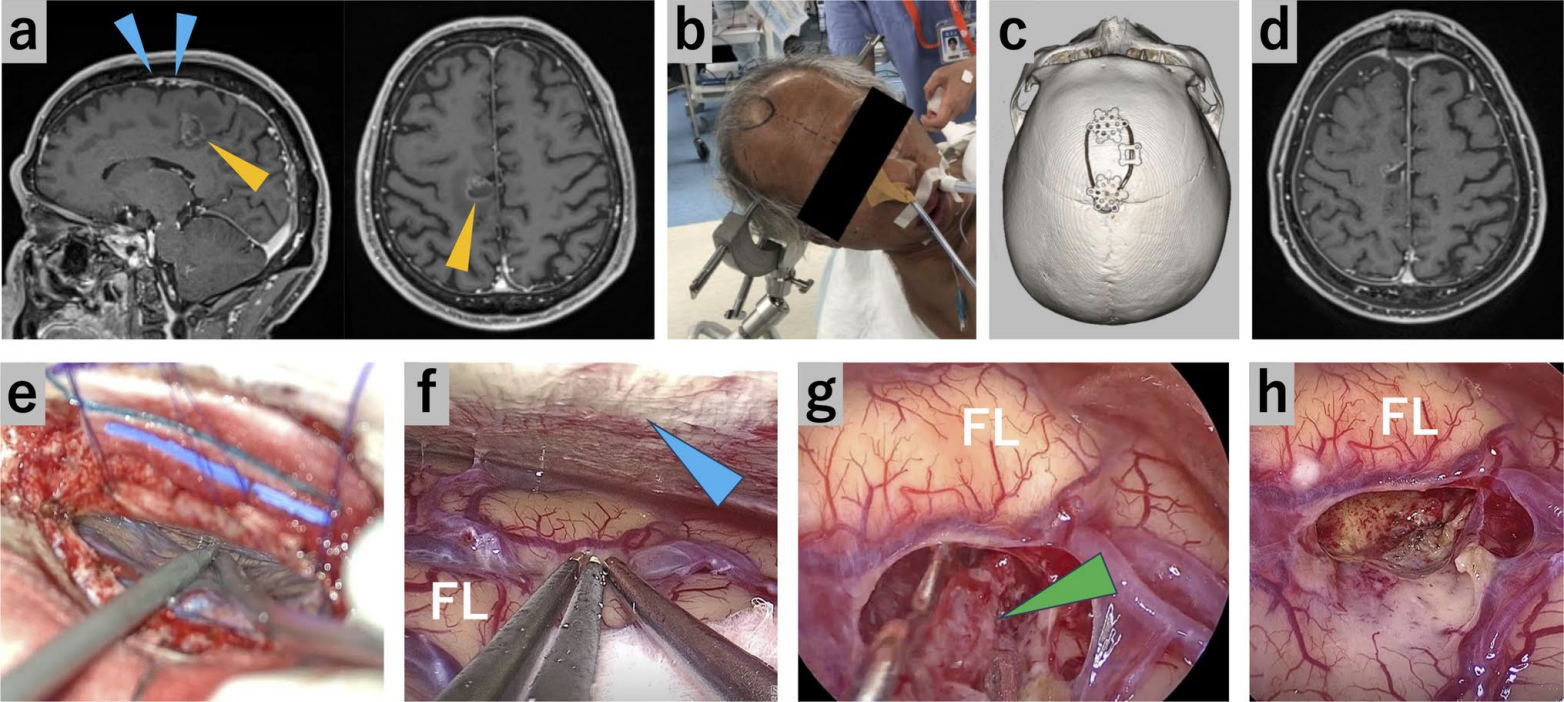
Case 1: Preoperative and postoperative radiologic images, surgical position, and intraoperative exoscopic and endoscopic photographs. (a) Preoperative MRI. The sagittal view shows well-developed bridging veins over the tumor. Yellow arrowhead, tumor; blue arrowheads, bridging veins. (b) Surgical position, with the head fixed in the supine lateral position and the affected side down. (c) Postoperative 3D-CTA, demonstrating craniotomy. (d) Postoperative MRI. (e) After dural incision, the major bridging veins above the tumor are not seen in the surgical view. (f) With CSF drainage, the endoscope is inserted into the interhemispheric fissure. Gravity-assisted brain retraction provides wide surgical fields. Blue arrowhead, the falx cerebri; FL, frontal lobe. (g) The tumor is resected under the endoscopic view. Green arrowhead, tumor; FL, frontal lobe. (h) After tumor resection. Hemostasis is performed under the endoscopic view. FL, frontal lobe
